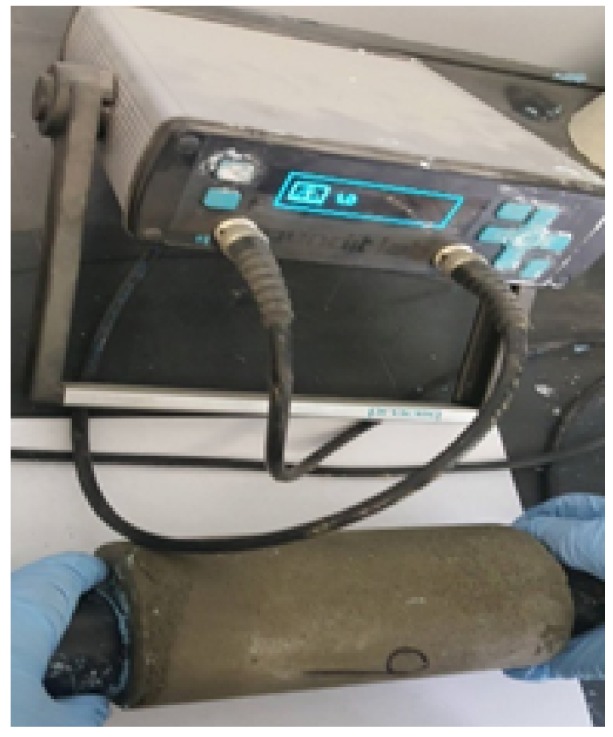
Ultrasound pulse velocity test setup.
